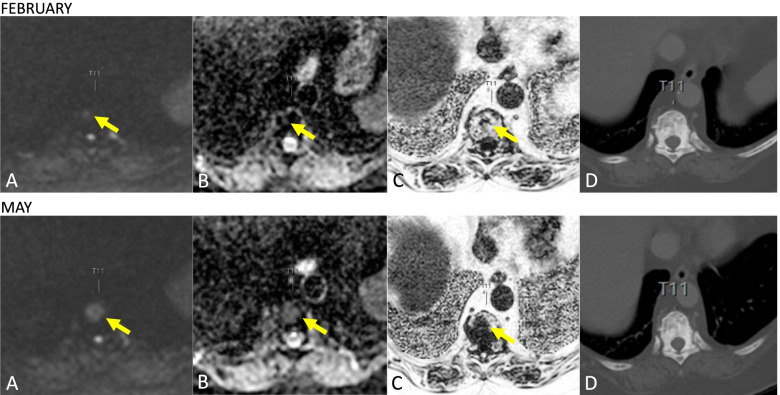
Progressive disease on WBMRI not identified on CT. 80-year-old female with ER- PR- positive HER-2 negative infiltrating ductal carcinoma with bone metastases on first presentation. Tumour marker rise was noted whilst on Letrozole and Denusomab over a three-month period. WBMRI demonstrated clear progression at several sites but no changes were appreciated on CT performed at the same time points. In this figure high b-value (b900) image (A), corresponding ADC map (B) and derived fat fraction images from the Dixon sequences (C) demonstrate an increase in the size of the lesion at T11 with no appreciable change in the mixed lytic and sclerotic disease burden on CT (D)
